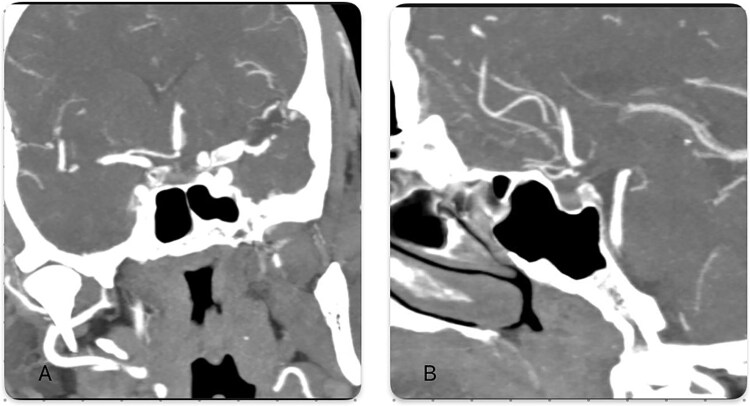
(A) Coronal computed tomography angiography (CT angiography) demonstrates the fusion of the bilateral anterior communicating arteries (A2) into a single midline pericallosal trunk (azygous anterior communicating artery [ACA]). The right anterior communicating artery (A1) is hypoplastic, and the dominant left anterior communicating artery supplies the trunk. A distal cutoff indicates occlusion. (B) Sagittal computed tomography angiography (CTA) reveals the midline ACA branching bilaterally, confirming the azygous configuration. Loss of distal opacification beyond the bifurcation suggests thrombotic occlusion.
